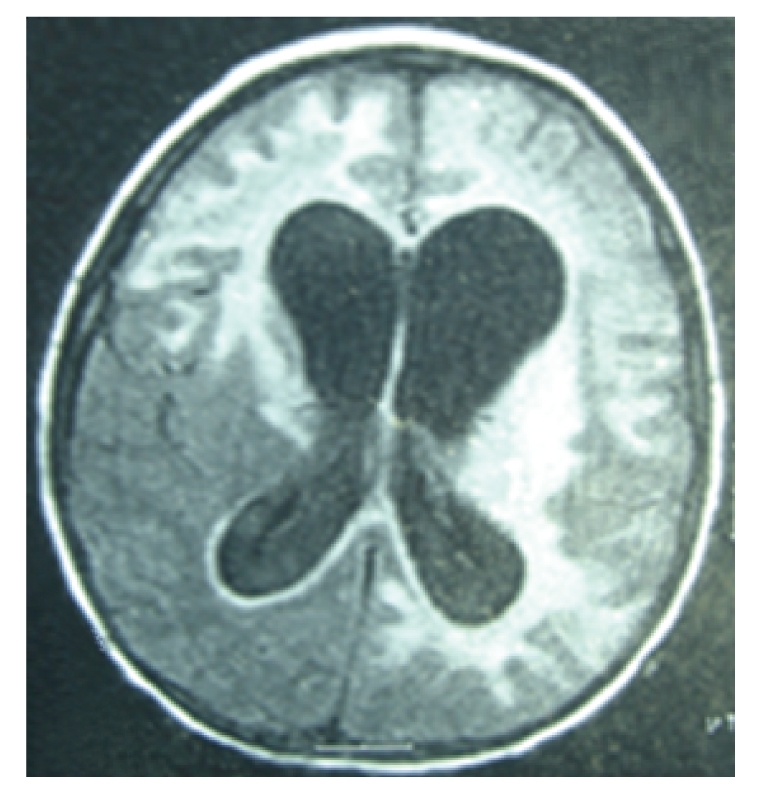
2nd MRI, 3 1/2 months after onset of symptoms, 3 days post-HAART cessation.
